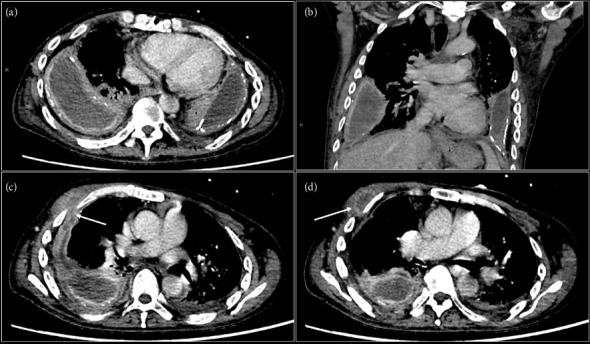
Contrast-enhanced computed tomographic images of the thorax in the portal venous phase, in axial (a) and coronal (b) planes, demonstrating the presence of bilateral pleural collections with thickened pleura and calcifications, which are features of chronic empyema. Consolidations are also seen in the lower lobes bilaterally. Axial images at different levels show the presence of a fistulous tract extending anteriorly from the right empyema (arrow, (c)), leading to a thick-walled collection in the right chest wall (arrow, (d)).
